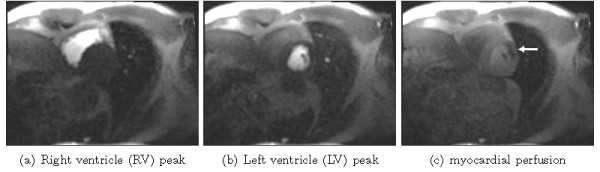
Images from a first-pass gadolinium-enhanced myocardial perfusion MRI study, here taken at the moment when the contrast agent first enters the right ventricle (RV) (a), then the left ventricle (LV) (b), and finally, perfuses the LV myocardium (c). Note, the hypointense region in the perfused myocardium (c) indicates a reduction in blood flow.
